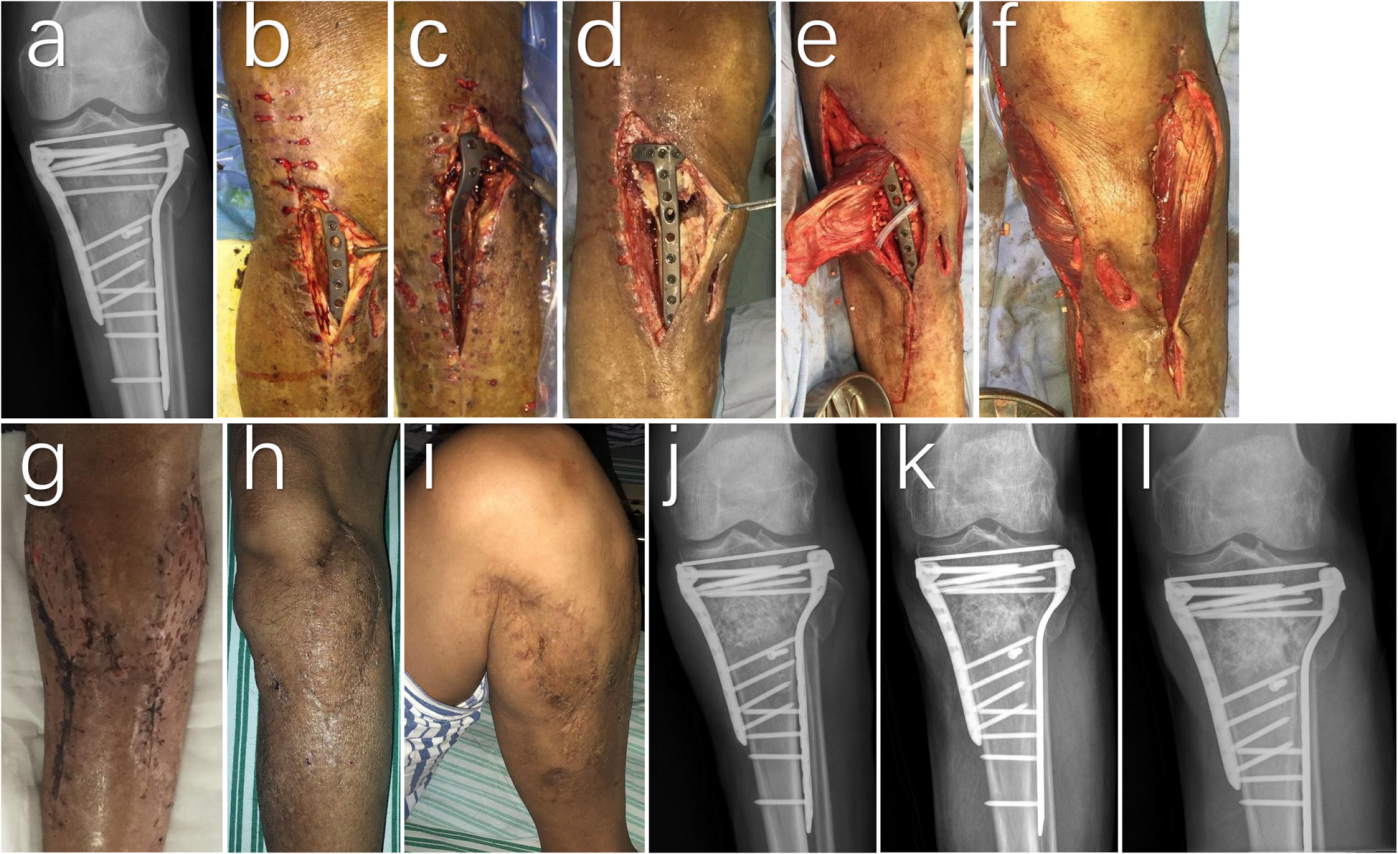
Typical case 1: Patient No. 14 presenting with plate exposure after tibial plateau fracture (Schatzker VI) surgery (b, c), The X-ray result shows the presence of local dead bone (a, red arrow). First, the necrotic tissue and inflammatory tissue (b, c) are completely removed. After removing the dead bone through the medial window, a 5 × 3 cm bone defect is found (d). The internal and external head muscle flaps of the gastrocnemius muscle are being designed and separated. The autologous iliac bone and the vancomycin/gentamicin-calcium sulfate-loaded artificial bone are being implanted into the bone defect (e). After covering the wound surface (f) of the leg with the internal and external cephalic muscle flaps from the gastrocnemius muscle, free skin grafting is performed on the surface of the muscle flap. Two weeks after operation, the skin graft survived and the incision healed well without swelling and exudation (g); The X-ray result shows that the bone graft was sufficient for the bone defect. The dead bone is also being removed (j). One year after operation, the patients recovered well (k). Two years after operation, the wound healed well and the knee joint flexion and extension function is good (h, i); The X-ray result shows that the fracture healed well (l)
